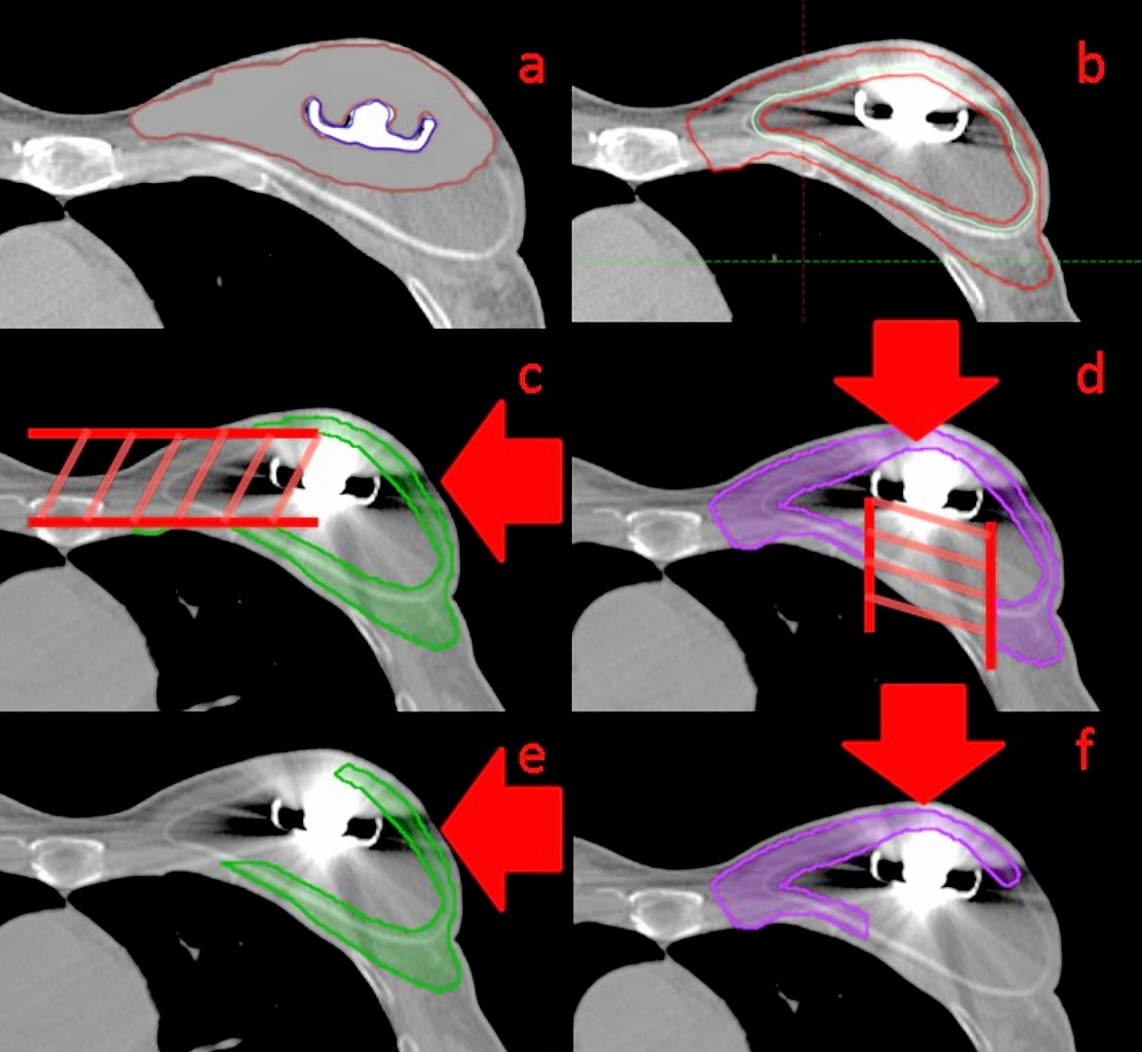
(a) The artifact override structure to account for CT artifact caused by the metal. (b) The contour of the implant (blue) and of the optimization target (red) which excludes all but a 0.3 cm rind of the implant. This is the target which is used in the optimization to apply prescription coverage. The field-specific targets for the lateral (c) and anterior (d) beams after excluding the section of the optimization target that is distal to the metal port with respect to the beam direction (indicated with red arrow). After defining a 3 cm overlap region through the center of the target where both beams will contribute dose, the final field-specific targets for planning for the lateral (e) and the anterior (f) beams are generated by excluding the section of the target beyond the overlap region with respect to the beam angle (field direction indicated with red arrow).
